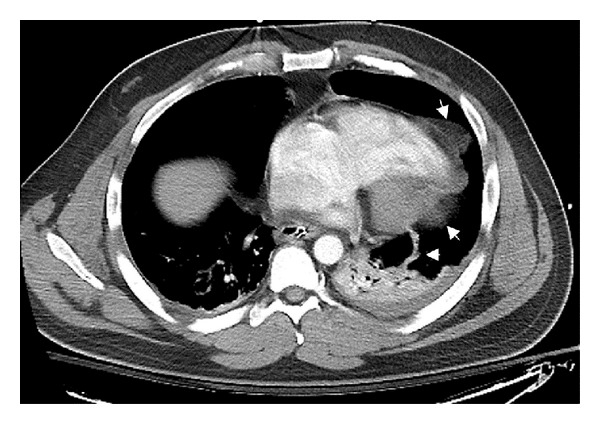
This CT study was performed shortly after arrival to the emergency room and shows the pericardium in an unnatural shape with the left ventricle herniating through the tear that runs vertically up the lateral aspect of the sac (white arrows).
